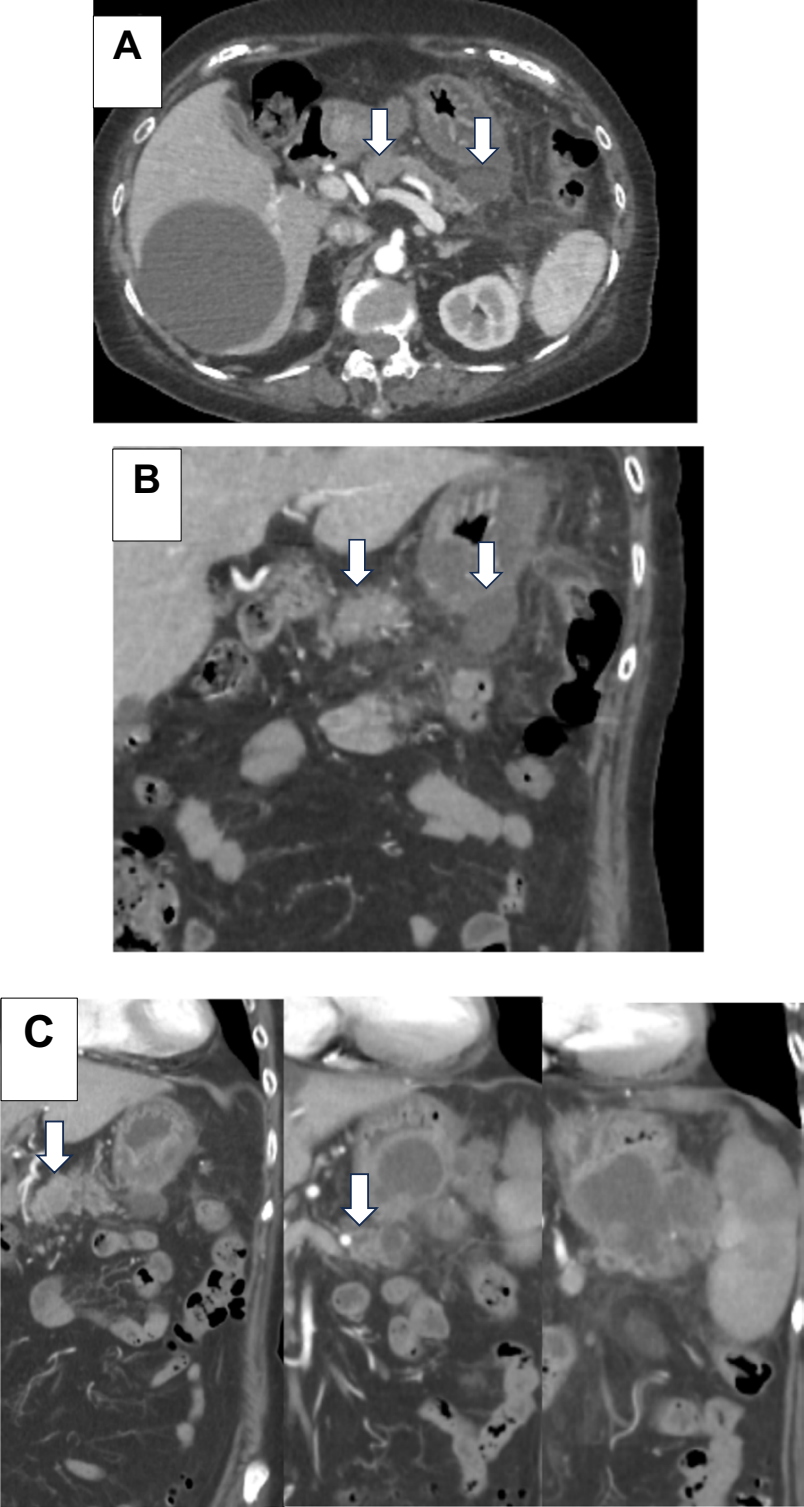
Abdominal dynamic CT findings. A–C CT revealed a 20-mm hypovascular tumor in the body of the pancreas and a 27-mm pseudocyst. The distal part of the main pancreatic duct was dilated due to obstruction by the tumor, and the pancreatic cyst was continuous with the pancreas (white arrow). *CT* computed tomography
